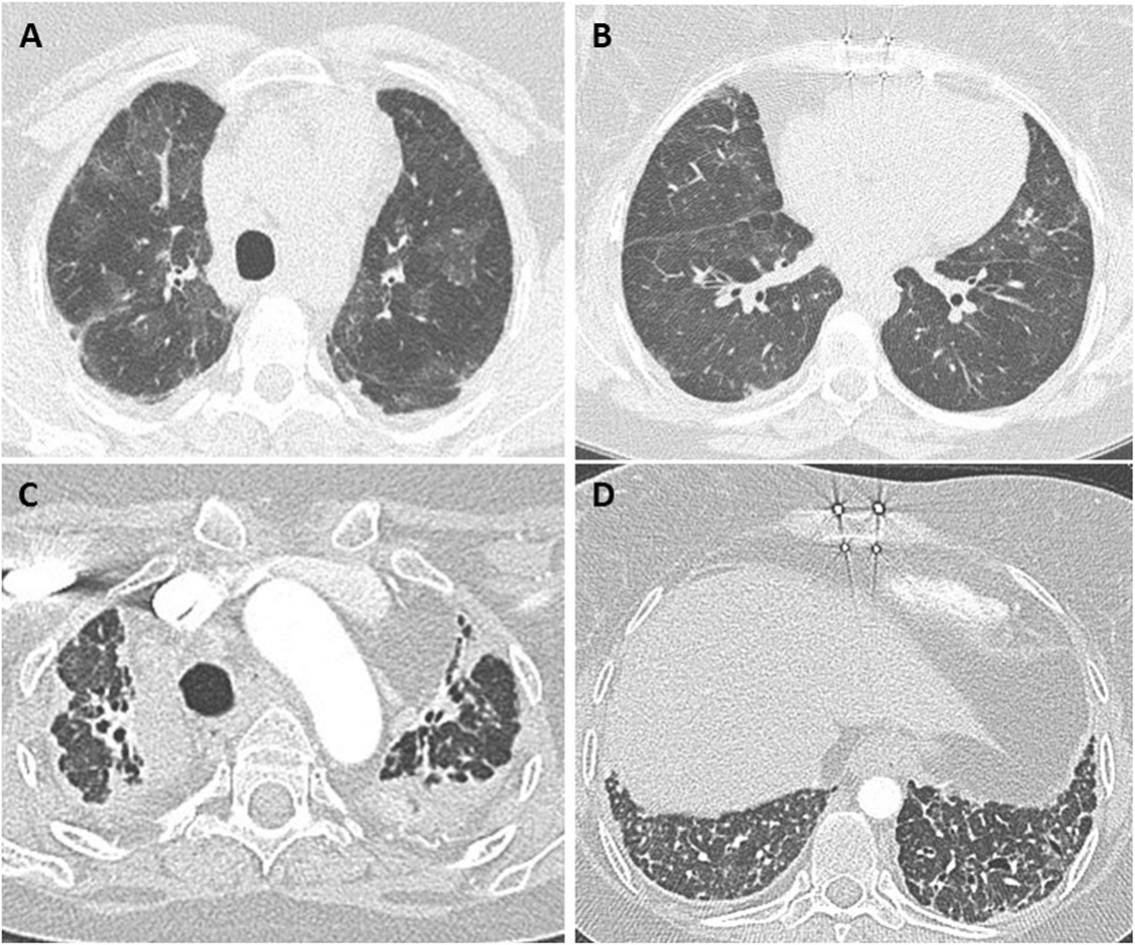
Imaging findings showing evolution of bronchiolitis obliterans to restrictive allograft syndrome. The patient initially presented with decreased FEV1 and mid-flows. Initial axial CT images (a and b) show mosaic attenuation, likely due to air-trapping. Transbronchial biopsy was negative for infection and acute rejection and the diagnosis of bronchiolitis obliterans syndrome was made. Follow-up CT images (c and d) after 3 years show evolution of changes with upper lobe predominant subpleural pleuroparenchymal fibrotic changes typical of restrictive allograft syndrome
